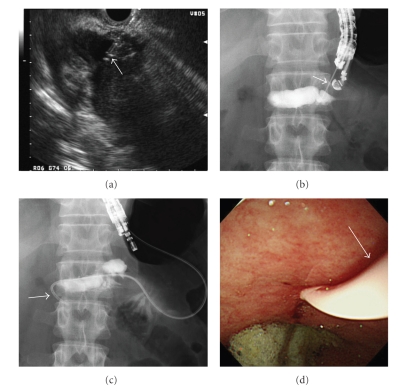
Endosonography-guided pancreatic duct drainage (ESPD): (a) endosonographic image, (b, c) X-ray image, and (d) endoscopic image. The PD was punctured via the body of the stomach with a 19-gauge needle (arrow) (a, b). A 7.2 F nasopancreatic duct drainage catheter (arrow) was placed (c, d).
